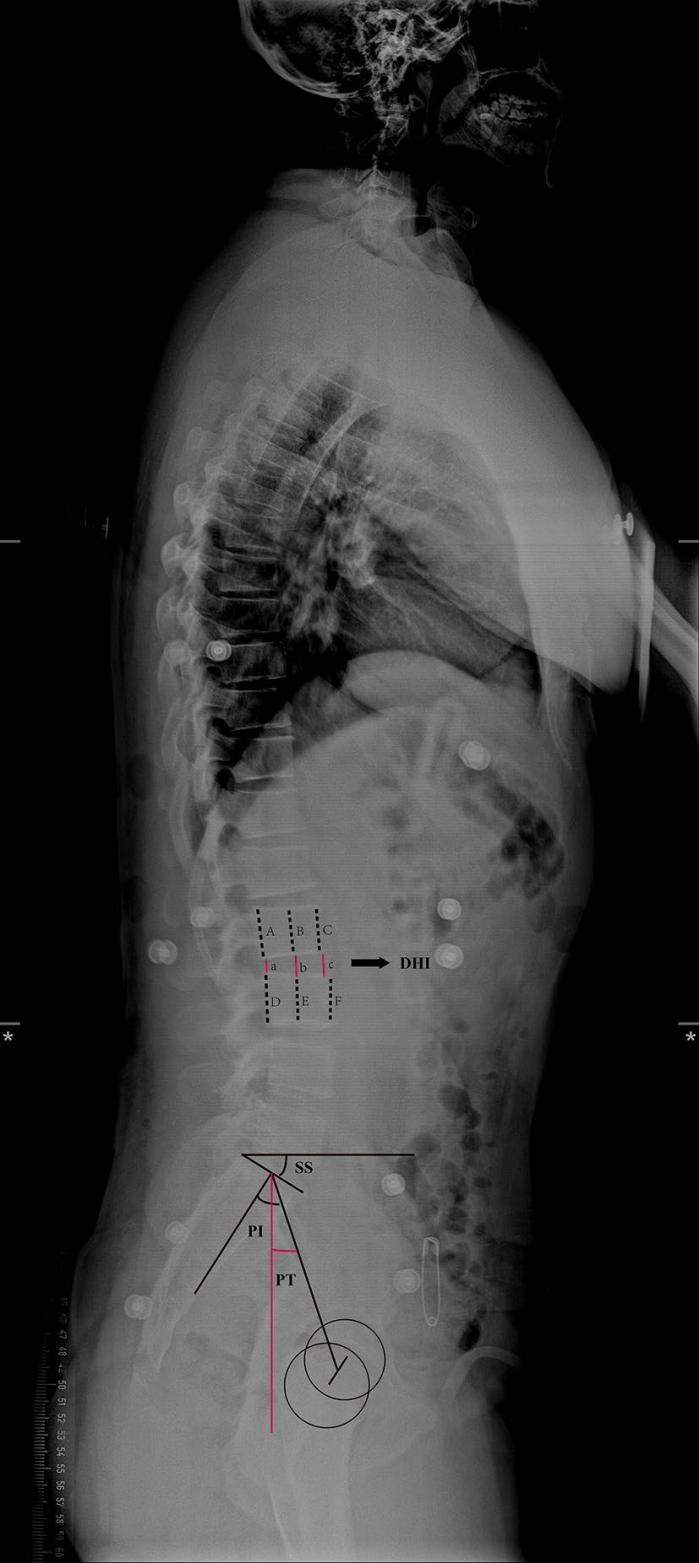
The definition of certain imaging parameters. Disc Height Index (DHI): The average of the anterior, middle, and posterior disc measurements was taken and it divided by the average of the heights of the adjacent upper and lower vertebral bodies, which were also the average of the heights of the anterior, middle, and posterior vertebral bodies. As shown in Fig. 1, DHI = (a + b + c)/(A + B + C + D + E + F)*2; Intervertebral Foramen Height (IFH): It was defined as the distance from the lowest point of the upper pedicle to the highest point of the lower pedicle on three-dimensional CT reconstruction; Range Of Motio (ROM): It was the angle that between the upper and lower endplate extension lines in the hyperextension position was subtracted from the angle between the upper and lower endplate extension lines in the hyperflexion position; Lumbar Lordosis (LL): It was the angle formed between the upper endplate of L1 and the upper endplate of S1; Pelvic Incidence (PI): It was defined as the angle between the line perpendicular to the sacral plate at its midpoint and the line connecting this point to the axis of the femoral heads; Sacral Slope (SS): It was defined as the angle between the superior plate of S1 and a horizontal line; Pelvic Tilt(PT): It was defined as the angle between the line connecting the midpoint of the sacral plate to the femoral heads axis and the vertical.
